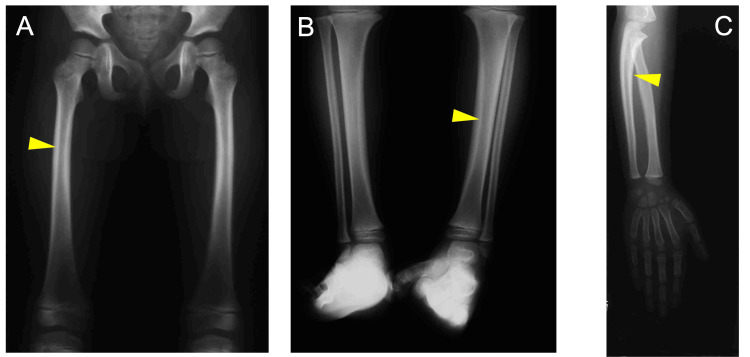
Radiographs of long bones at age three A: Femur. B: Tibia. C: Ulna. Yellow arrowheads indicate thickening of the cortices of limb bones
